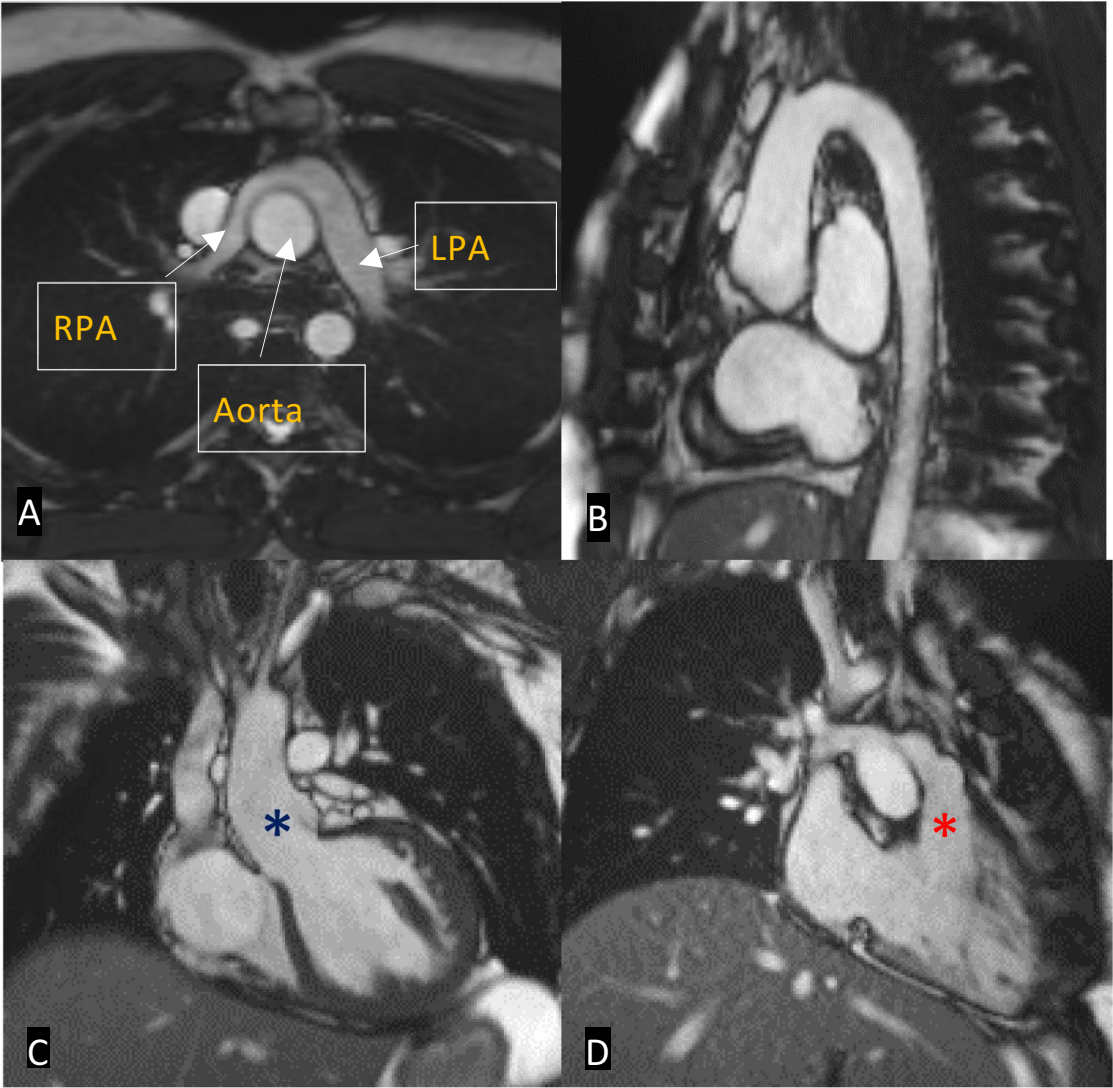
Still shots of MRI SSFP cine in axial (A), sagittal oblique (B), coronal left ventricular outflow tract (C), and right ventricular inflow and outflow (D) views post-arterial switch for D-TGA. A Typical appearances of the Lecompte manoeuvre for the Jatene procedure. The pulmonary arteries bifurcate anterior to the ascending aorta, classically ‘draping’ over it. B Typical appearances of a high aortic arch. C Dilatation of the neo-aortic root (blue asterisk). D Normal appearances of the neo-pulmonary root (red asterisk). RPA: right pulmonary artery; LPA: left pulmonary artery
